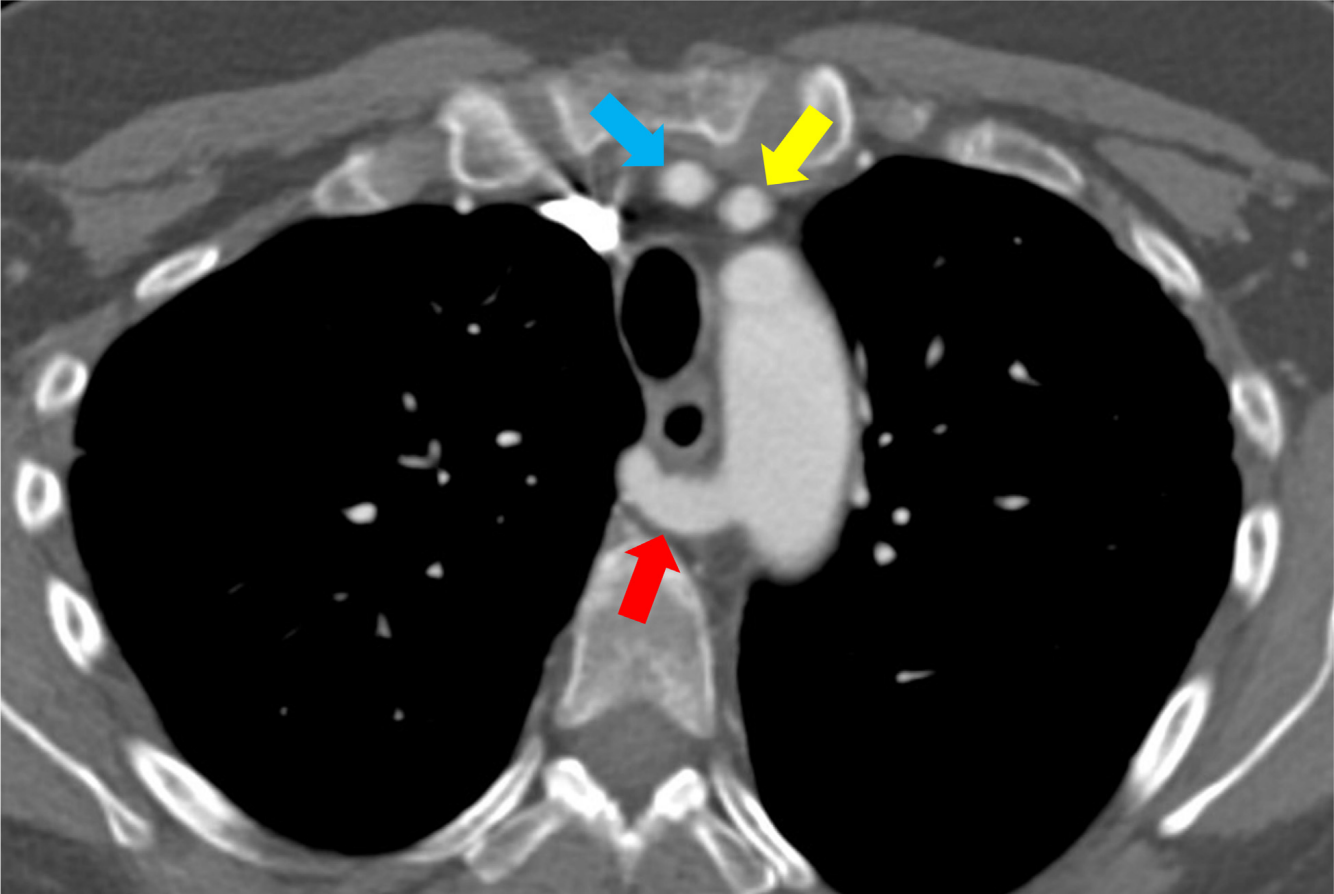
ARSA: Axial CT with contrast at the level of the aortic arch shows the right subclavian artery (red arrow) arising from the distal aortic arch and crossing the midline behind the esophagus. Also seen is the right common carotid artery (blue arrow) and left common carotid artery (yellow arrow) which are the 1st and 2nd branches from the aortic arch in the setting of an ARSA.
